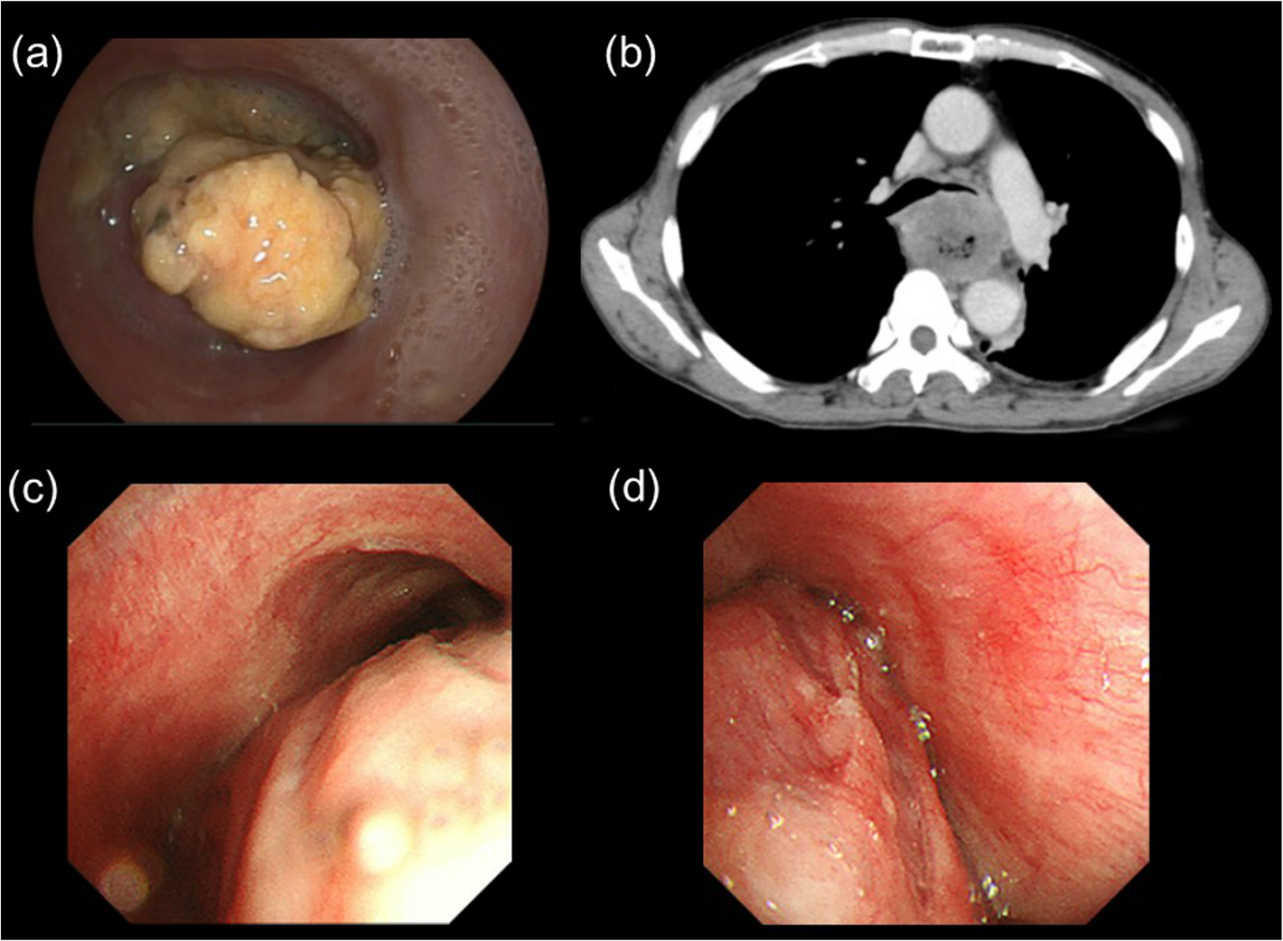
a Esophagogastroduodenoscopy revealed that the esophageal lumen was filled with necrotic components from the primary esophageal cancer; b computed tomography (CT) revealed that the left bronchus was compressed by the primary esophageal tumor components; c bronchoscopy revealed that the tracheal lumen at the carina level was deformed by the primary esophageal tumor components; d bronchoscopy revealed that the left bronchus presented with an almost complete obstruction due to compression by the primary esophageal tumor components
